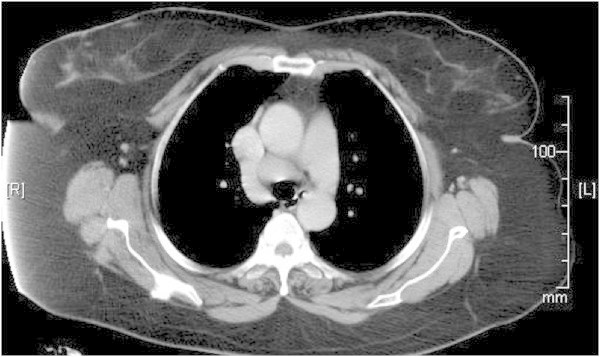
Computed tomography showing mediastinal lymphadenopathy.
